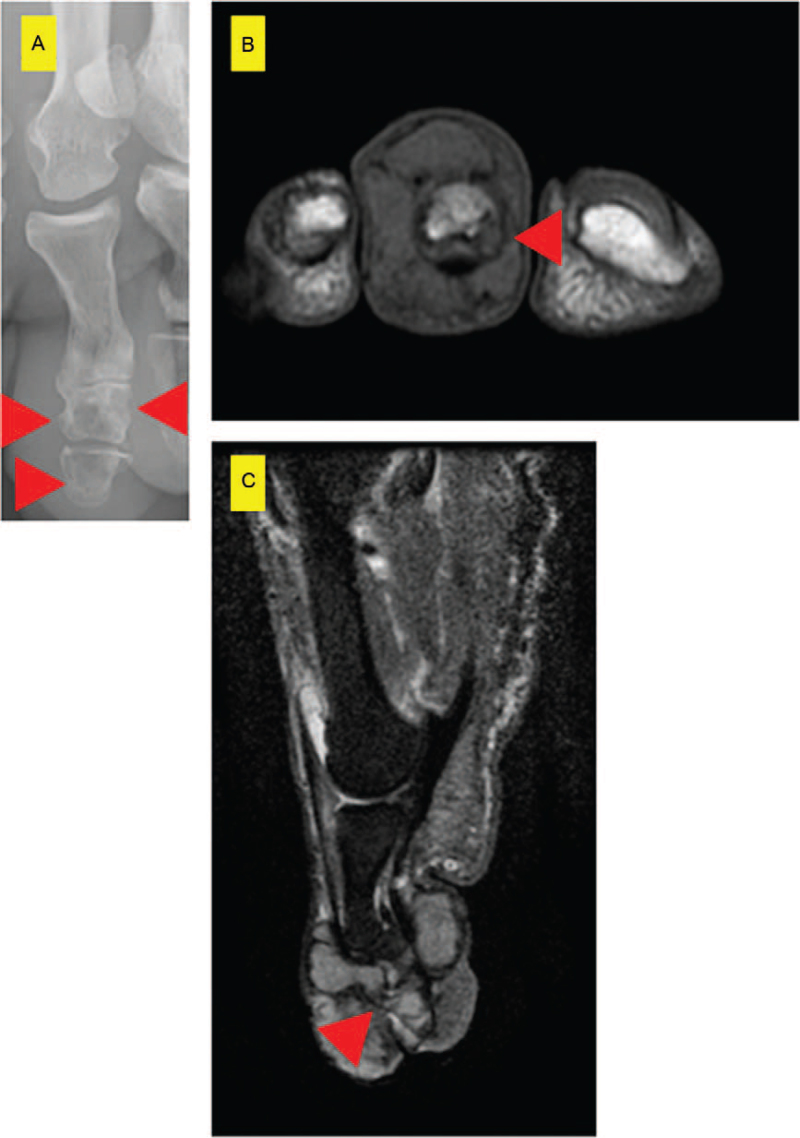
(A) The radiograph showed a soft tissue shadow enhancement at the distal end of the second toe and lytic lesions (red arrowheads) in the intermediate and distal phalanges. (B) Coronal-view magnetic resonance imaging (MRI) revealed a low-intensity mass surrounding the second toe. Partial invasion of the tumor into the bone was observed. Red arrowheads indicate lytic lesions in the bones. (C) Sagittal-view MRI revealed a high-intensity mass surrounding the second toe. Partial invasion of the tumor into the bone was observed. Red arrowheads indicate lytic lesions in the bones.
